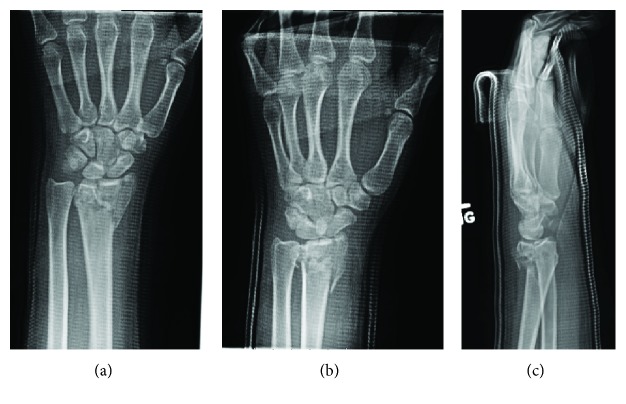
Radiographic presentation. Initial imaging on presentation to hand clinic after the patient was splinted at another facility.
